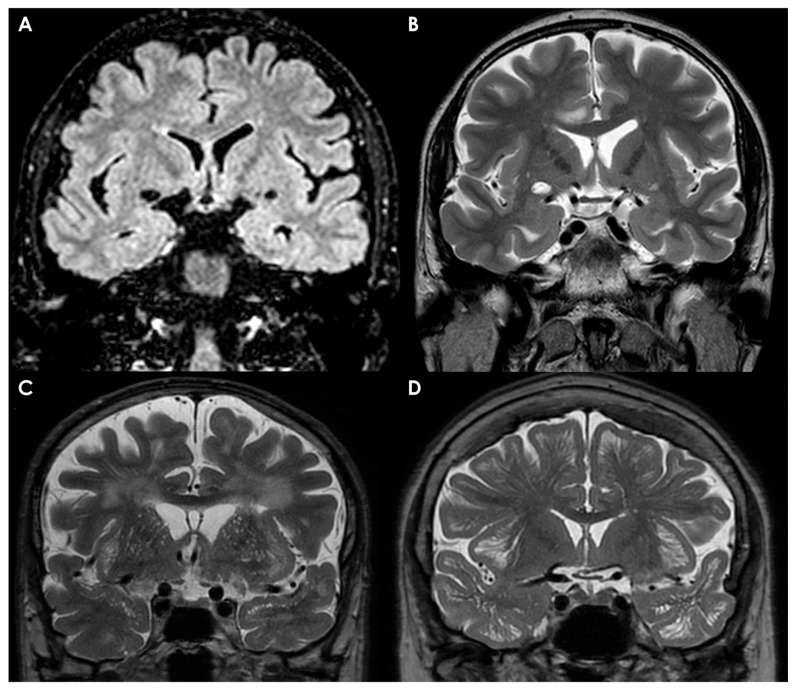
Brain MRI of different subtypes of EPVSs. In panel (A) (coronal FLAIR) and (B) (coronal T2), a large PVS in the right basal ganglia location (under the lenticular nucleus) is evident. In panels (C,D), there are two different examples in coronal T2, showing multiple deep (panel (C)) and lobar (panel (D)) EPVSs.
